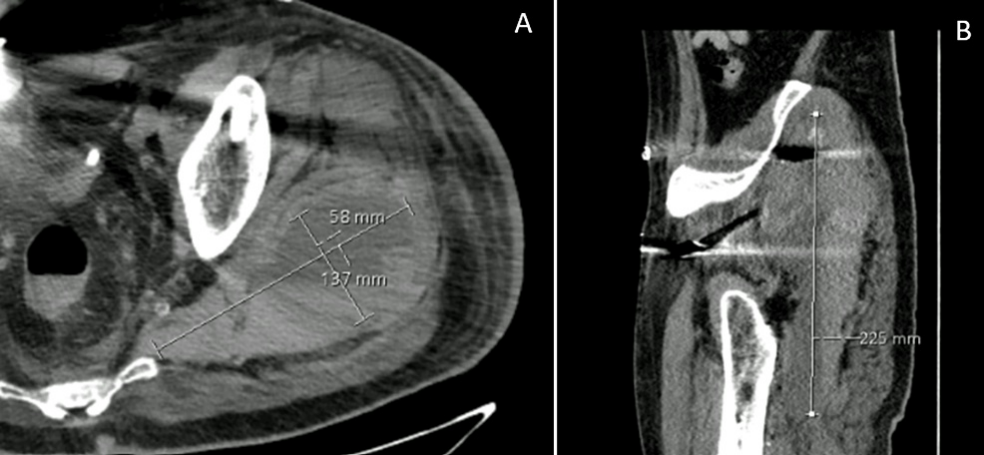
Axial (A) and sagittal (B) CT scans showing a large hematoma in the left gluteal region
